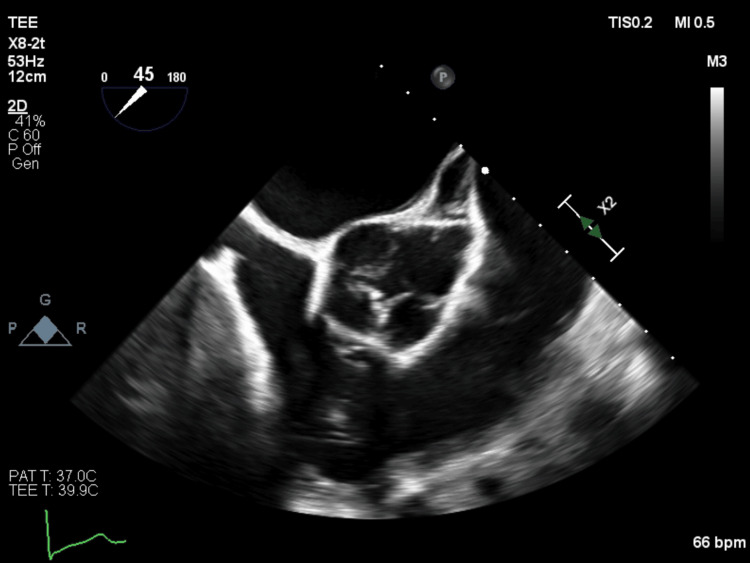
Quadricuspid aortic valve evidenced in short axis view on transesophageal echocardiogram (TEE).
